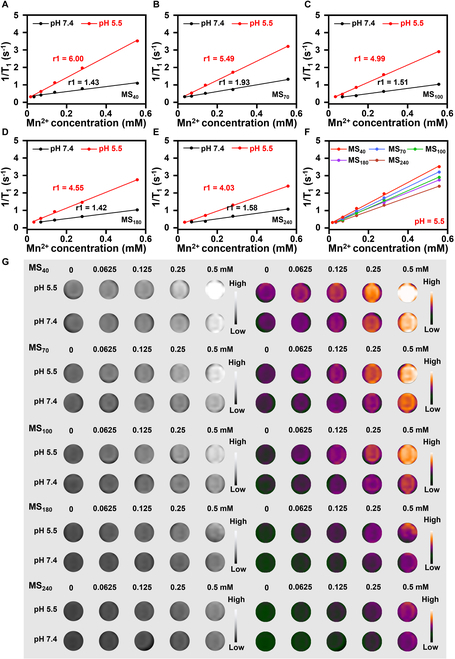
MRI performance of MnS@PAA with different sizes. (A to E) T_1_ relaxation rates of MnS@PAA with different sizes under various pH conditions from a 0.5-T MRI system in vitro. (F) T_1_ relaxation rates of MnS@PAA with different sizes in the same acidic environment (pH 5.5) in vitro. (G) T_1_-weighted MR image of MnS@PAA with different sizes from a 3.0-T MRI system.
